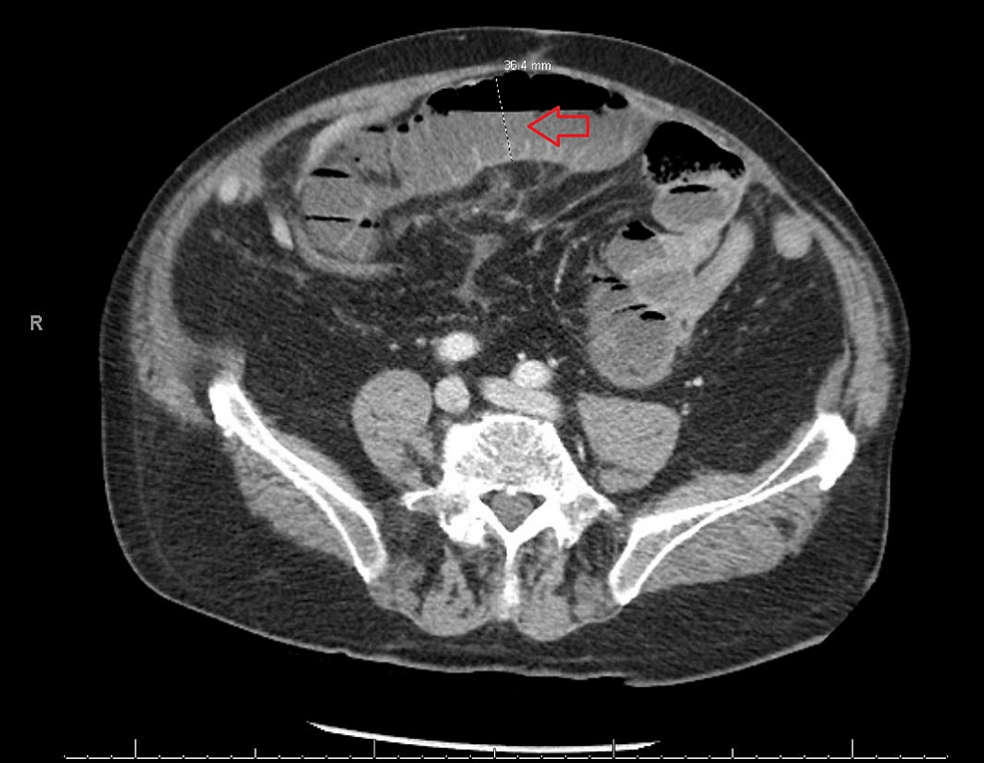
CT scan of the abdomen. Red arrow: Dilated small bowel loop, 4 cm in diameter.
